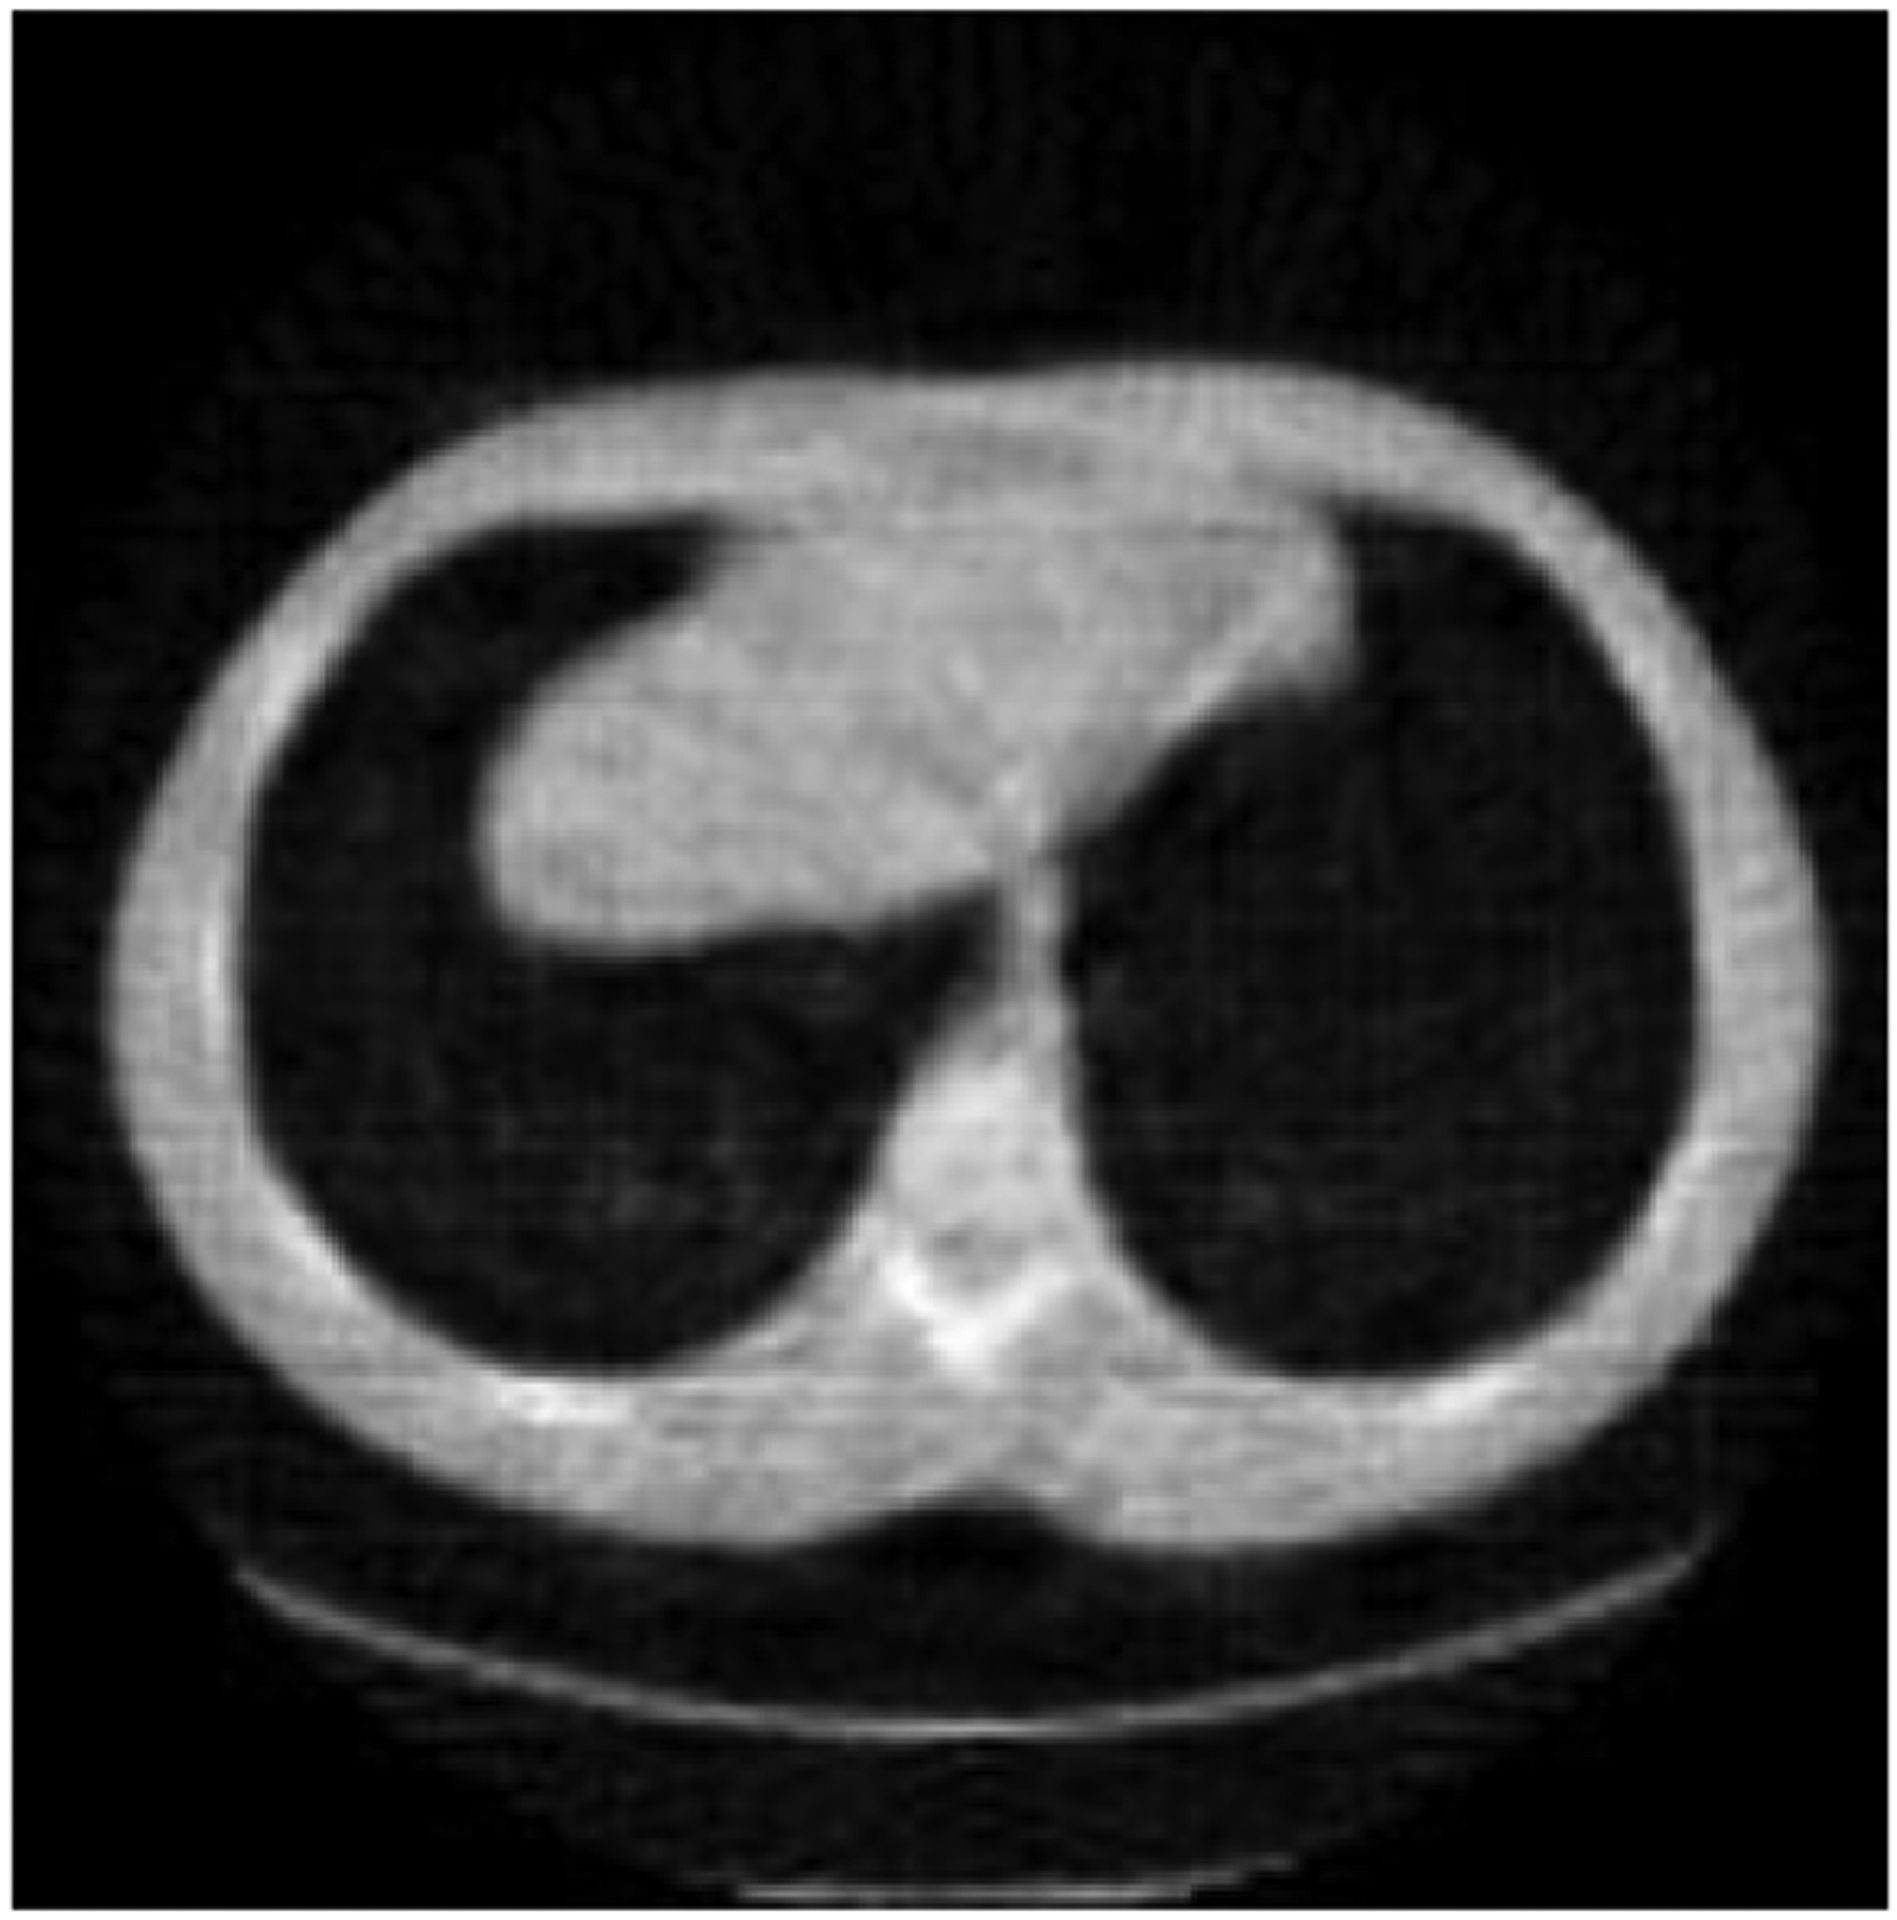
Reconstruction from model-interpolated sonogram.
